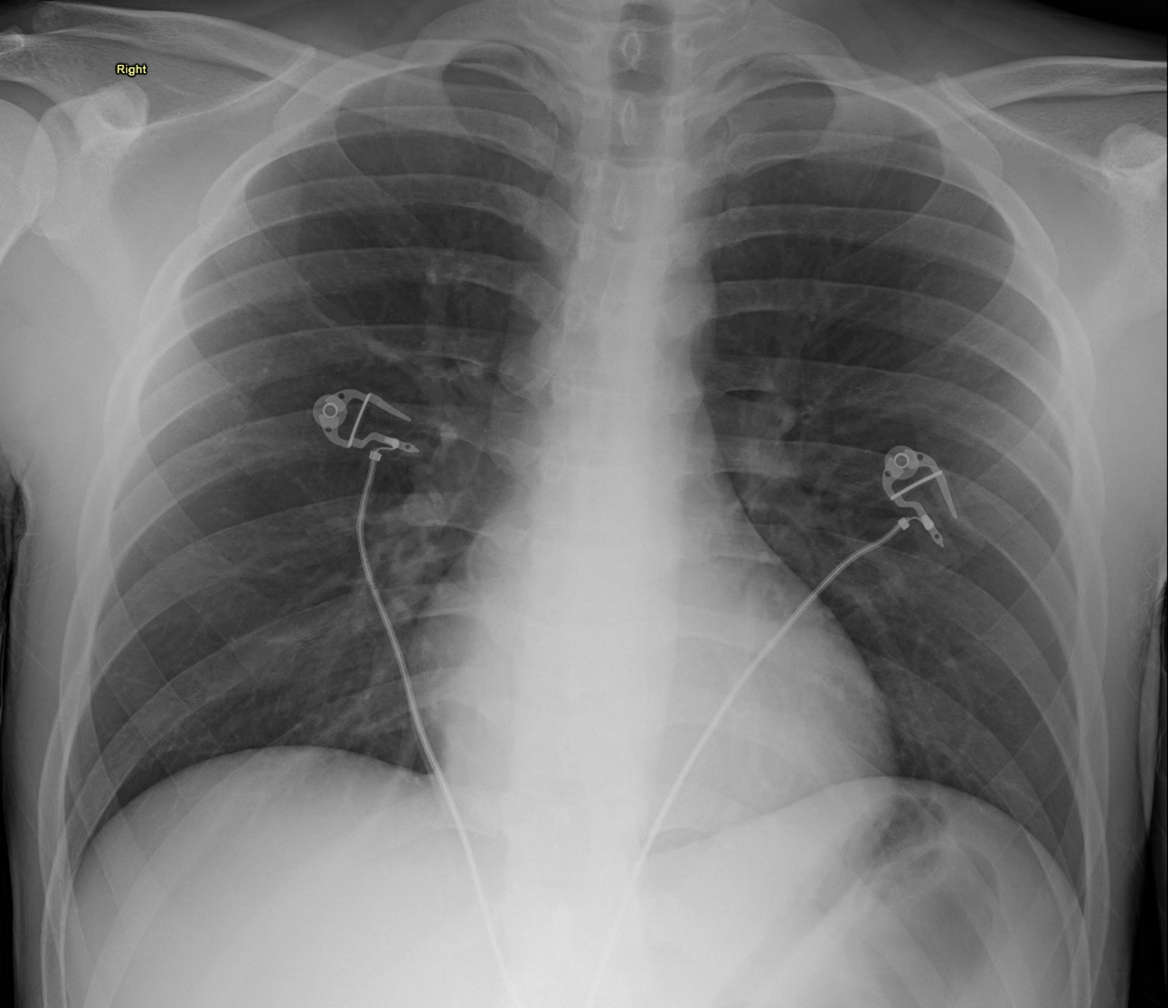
Chest X-ray shows no focal infiltrates, well-inflated lungs
